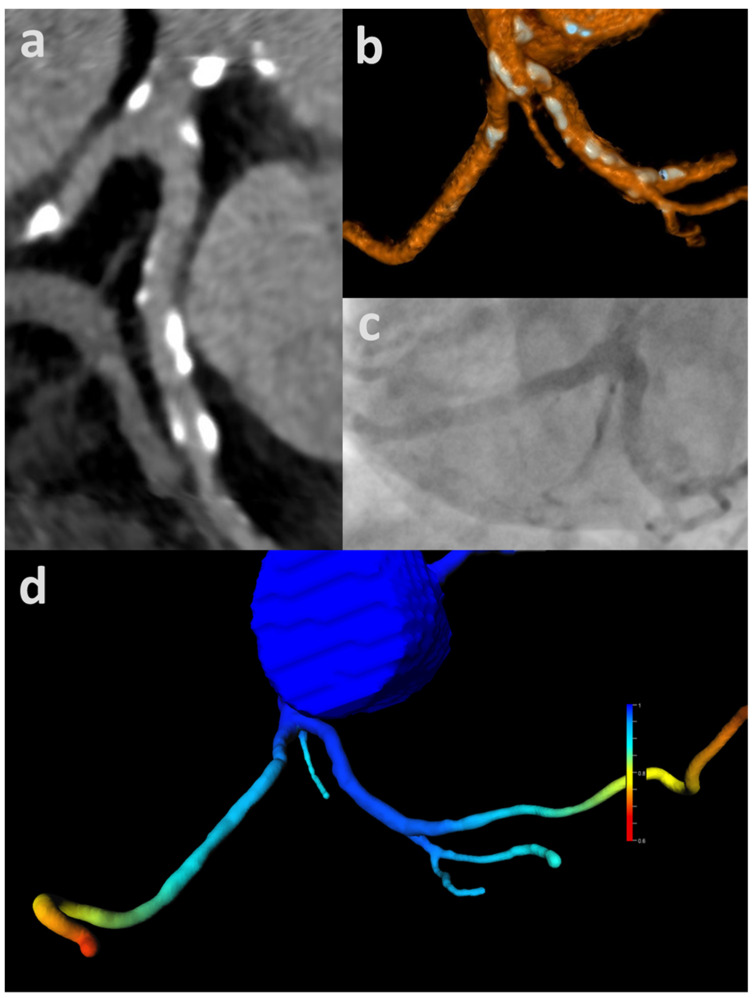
CT-FFR confirming negative cCTA: Heavily calcified left coronary artery (total CAC = 1834 AU) with trifurcation into left anterior descending (LAD), left circumflex (LCX) and intermediate artery without luminal obstruction depicted on cCTA as curved multiplanar reformation (a) and volume-rendered technique (b). The corresponding projection of invasive coronary angiography shows no stenosis (c). CT-FFR shows normal values well above 0.80 up to the distal vessels with a physiological drop-off of values only in the most distal runoffs (d). CAC—coronary artery calcium score; cCTA—coronary CT-angiography; CT-FFR—CT-derived fractional flow reserve. Adapted with permission from Gohmann et al. [8].
